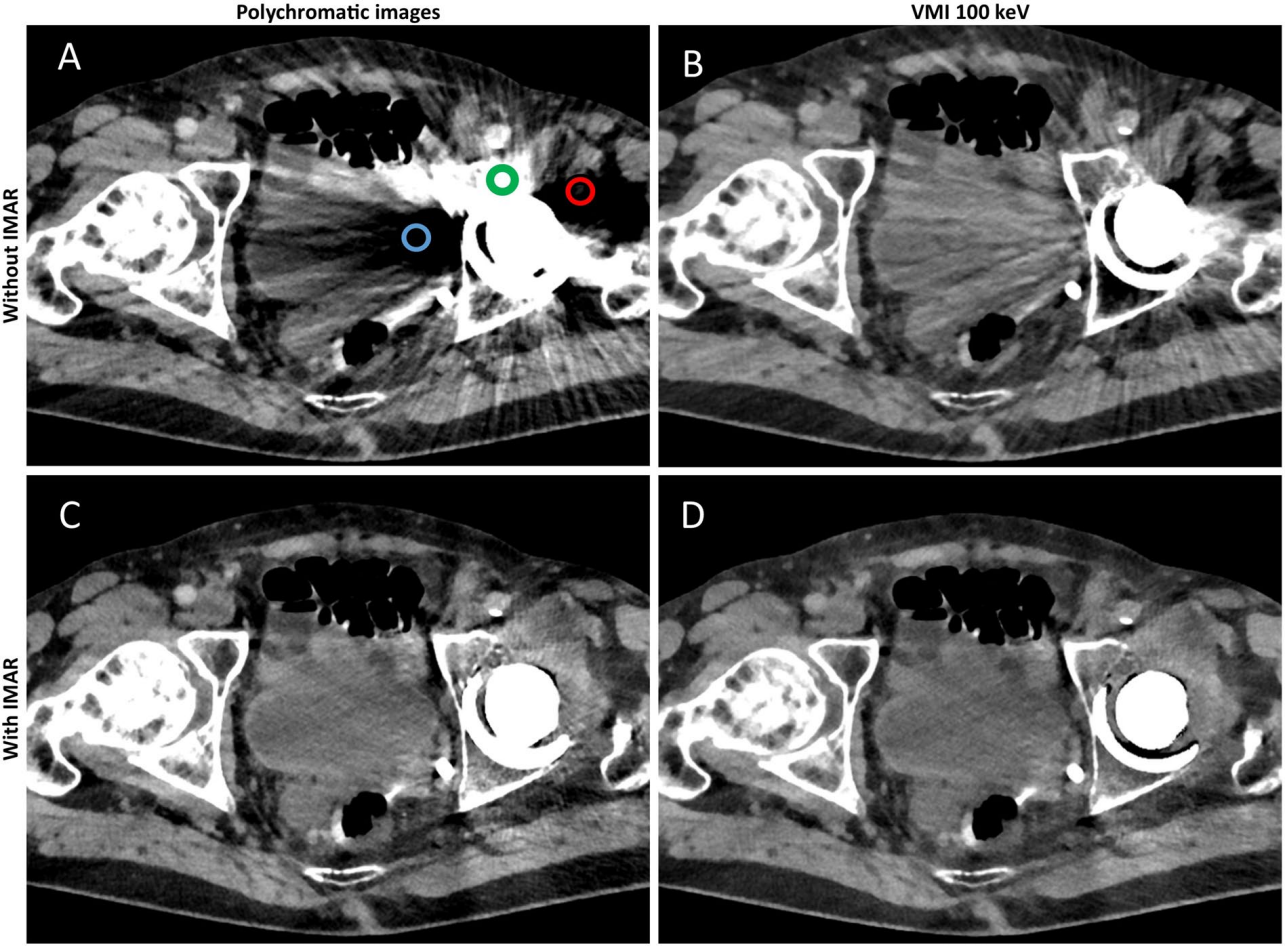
Representative axial polychromatic image (A), virtual monoenergetic image (VMI) of 100 keV (B), polychromatic image with iterative metal artifact reduction (IMAR) (C), and VMI of 100 keV in combination with IMAR (D) acquired with a photon-counting detector CT (PCD-CT) (window width/window level: 300/40 HU). Examples of ROI-Placement for artifacts in bone tissue (green), muscle tissue (red) and bladder tissue (blue) are shown in A. ROIs for corresponding artifact-free tissue are placed in slices in corresponding tissue without artifacts. Artifacts caused by hip prosthesis are most sufficiently reduced with the combination of VMI and IMAR.
